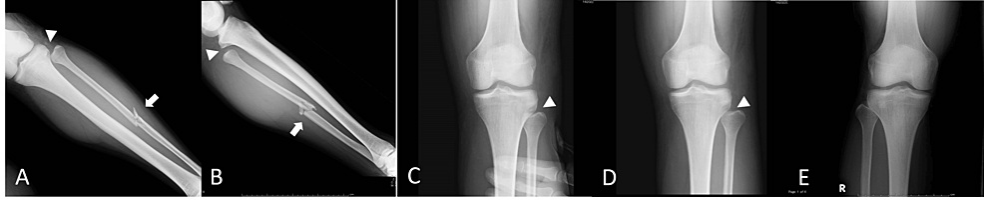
Initial X-ray radiographs and fluoroscopic findings (A, B) Initial X-ray radiographs (anteroposterior (A) and lateral view (B)). A fracture at the fibular diaphysis is diagnosed (white arrow), but the dislocation of the proximal tibiofibular joint is neglected (white arrowhead). (C, D, E) Fluoroscopic findings. Under fluoroscopic guidance, the dislocated fibula head of the left knee is reduced manually (C); however, it is easily re-dislocated (D); right knee anteroposterior view (E).
